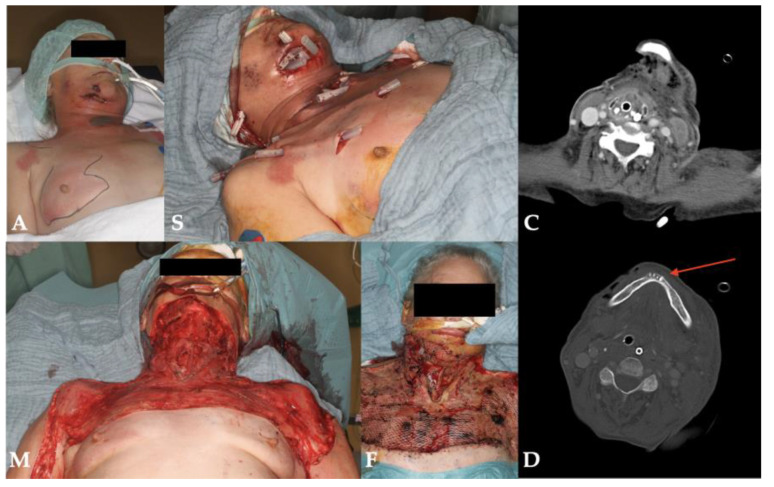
Clinical course and radiographs of patient No. 2: A: admission; S: surgery; M: maximal extent; F: final result; C: CT scan; D: dental focus (lower incisors).
